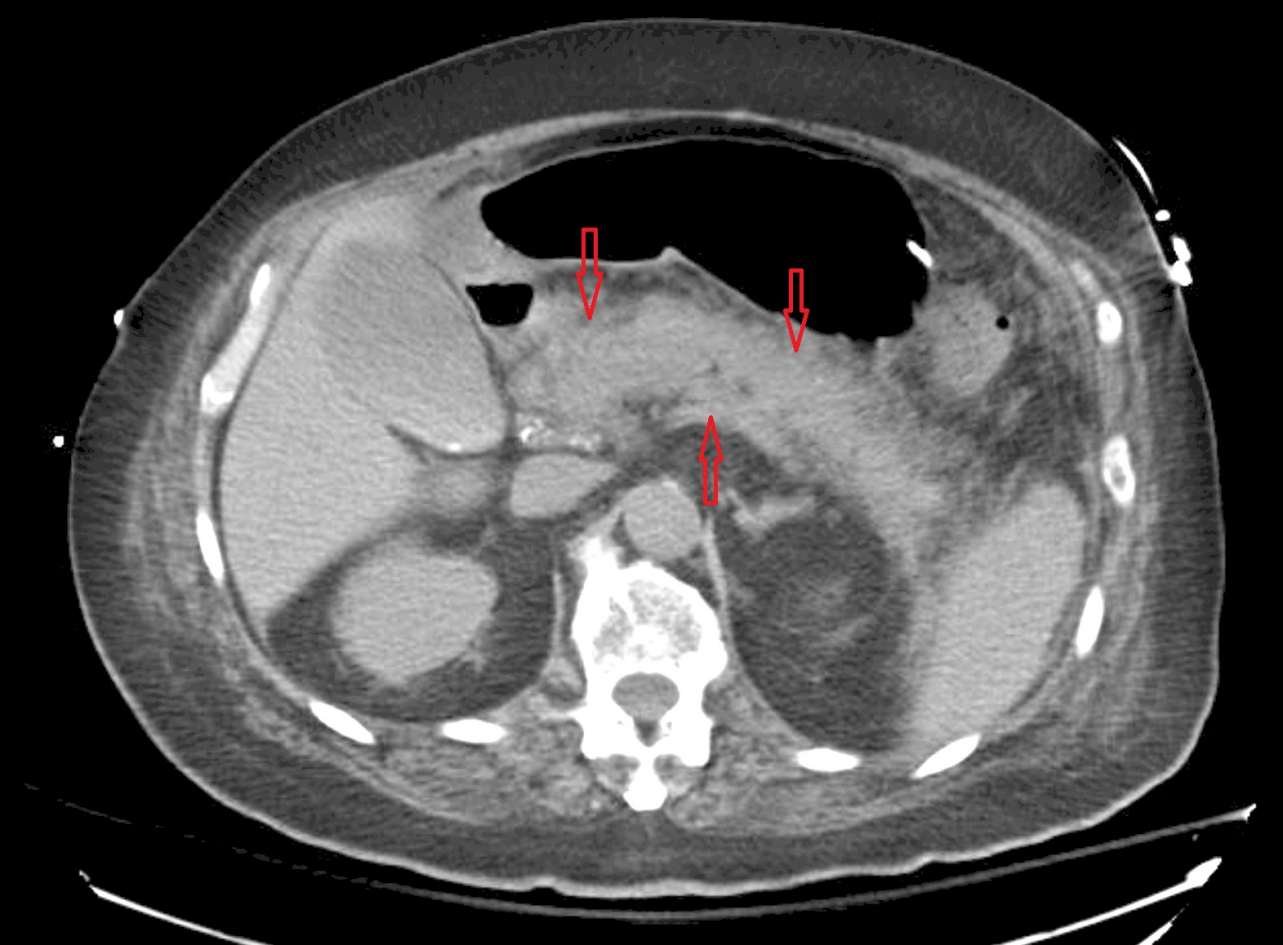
Acute pancreatitis. Computed tomography (CT) of abdomen demonstrating diffusely enlarged pancreas with indistinct margin due to inflammation representing acute pancreatitis (red arrows pointing towards inflamed pancreas).
